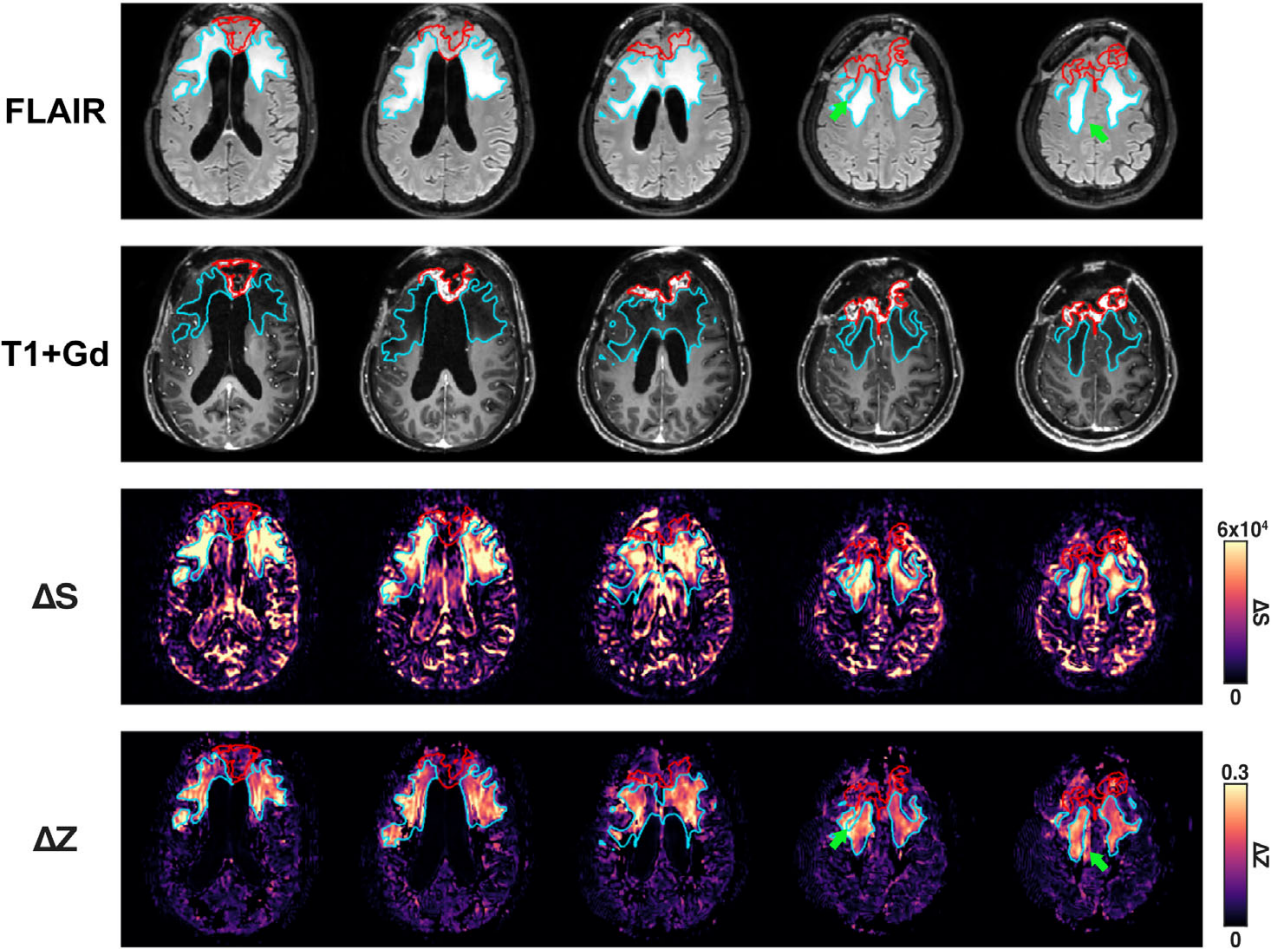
FLAIR, post‐contrast T1w, MISL ΔS, and MISL ΔZ images of a patient with brain metastases. The tumor is outlined in red and the peritumoral edema in blue. Green arrows indicate regions not hyperintense on FLAIR but showing elevated MISL ΔZ.
